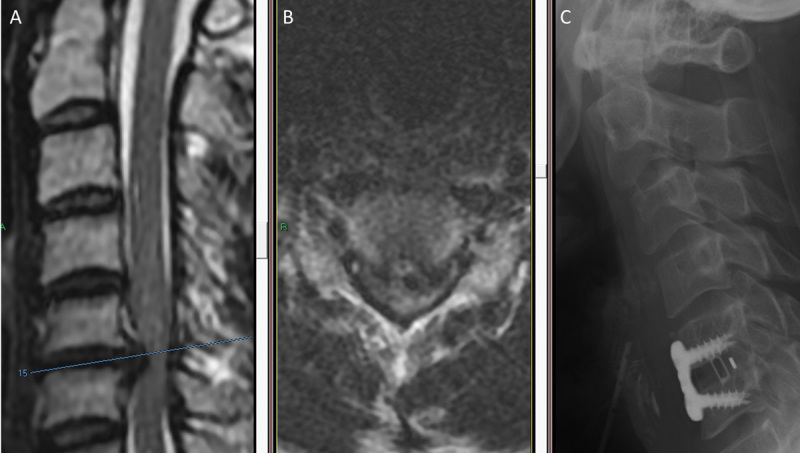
Acute disk herniation caused acute quadriparesis that rapidly progressed to quadriplegia during magnetic resonance imaging (MRI) examination in this 34-year-old man. (A) Sagittal and (B) axial T2-weighted MRI examinations demonstrate a central C5–C6 disk herniation with severe spinal cord compression. (C) Lateral X-ray demonstrates C5–C6 diskectomy and fusion with implantation of polyetheretherketone cage and fixed titanium plate.
